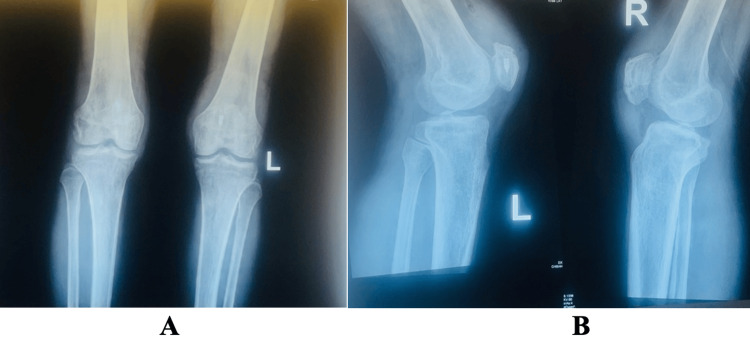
Radiographs of bilateral patellae (anteroposterior and lateral views) demonstrating healed patellae
